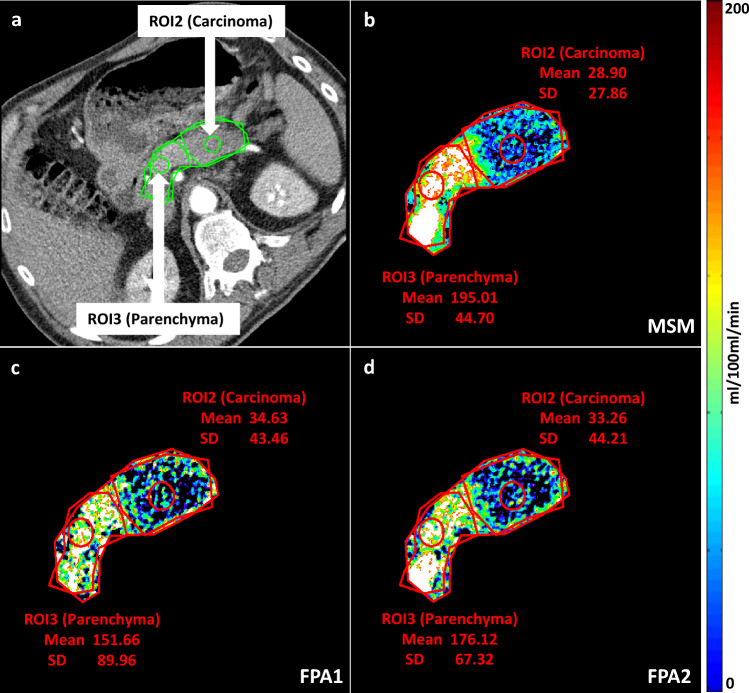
First pass analysis (FPA) and maximum slope model (MSM) perfusion maps. Conventional dynamic CT image (a), MSM perfusion map (b), FPA1 perfusion map at t_max_ (c) and FPA2 perfusion map at t9 (d) are shown for an adenocarcinoma patient. The blood flow (BF) perfusion maps show mean and SD values for circular ROI2 (carcinoma) and ROI3 (parenchyma). Note the higher mean BF of parenchyma tissue as compared to carcinoma for FPA1, FPA2, and MSM. Mean values are comparable between FPA1, FPA2, and MSM for the corresponding tissue regions.
